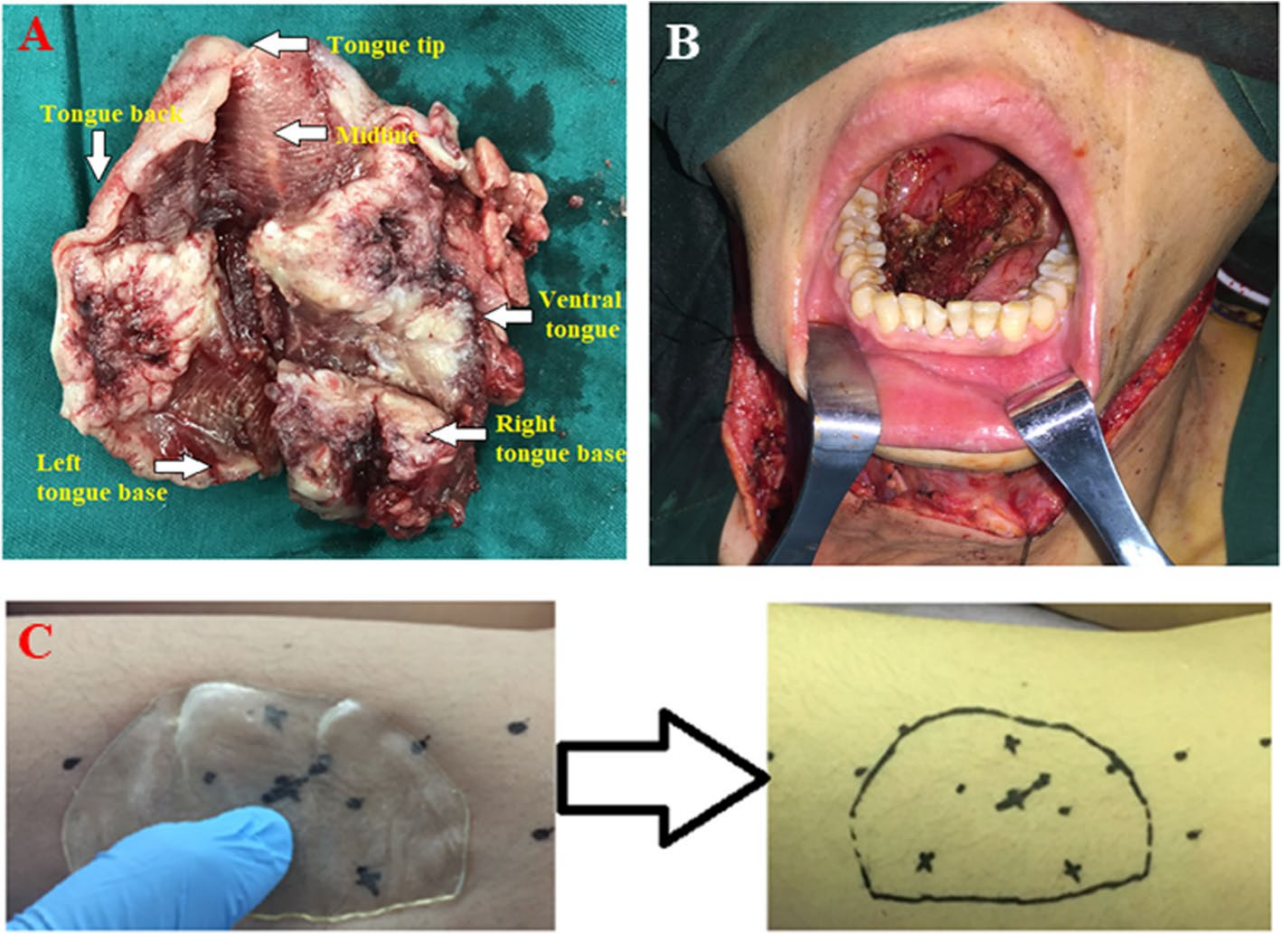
Surgical resection of the tumor and flap preparation. A The resected tumor. B Retained left tongue base. C Flap perforator blood vessels were located by preoperative Doppler ultrasound and flap perforator was marked with “X”. The surface area was defined by the 3D-printed flap cutting guide plate and with the “X” as its center
